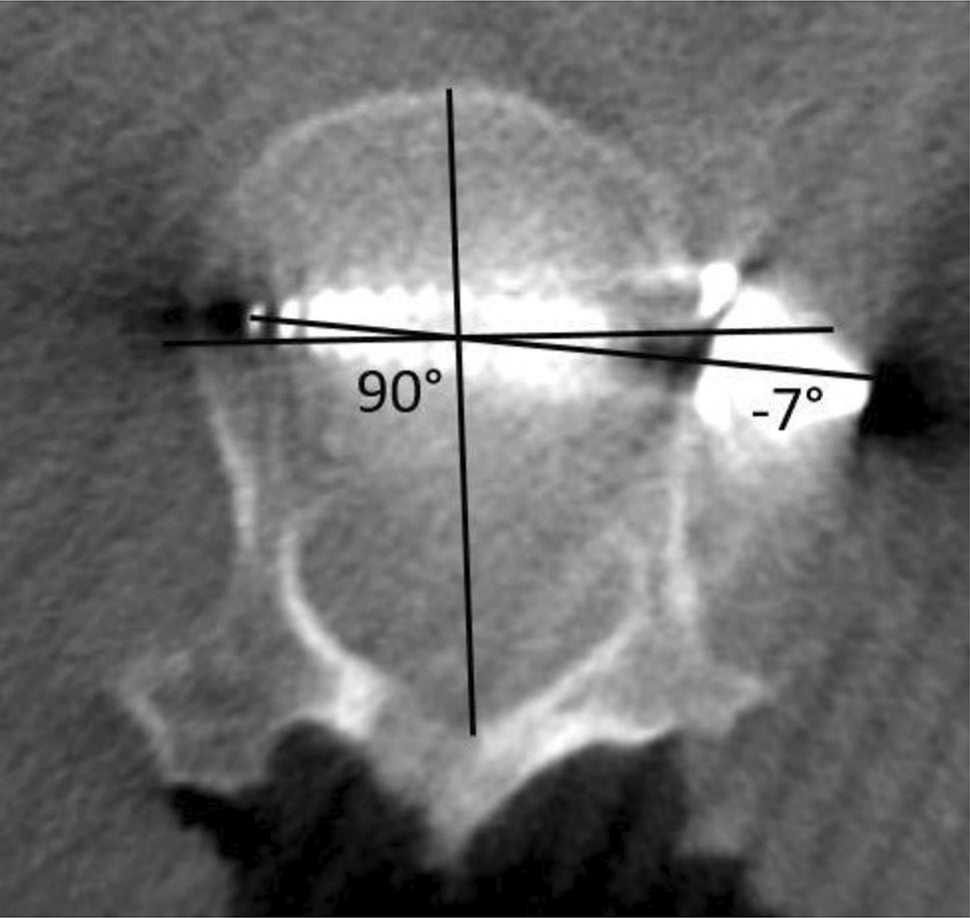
Axial CT image showing a line perpendicular to the long axis of the vertebral body, and a line through the long axis of the visualized screw with a screw angle of − 7° posterior to anterior
